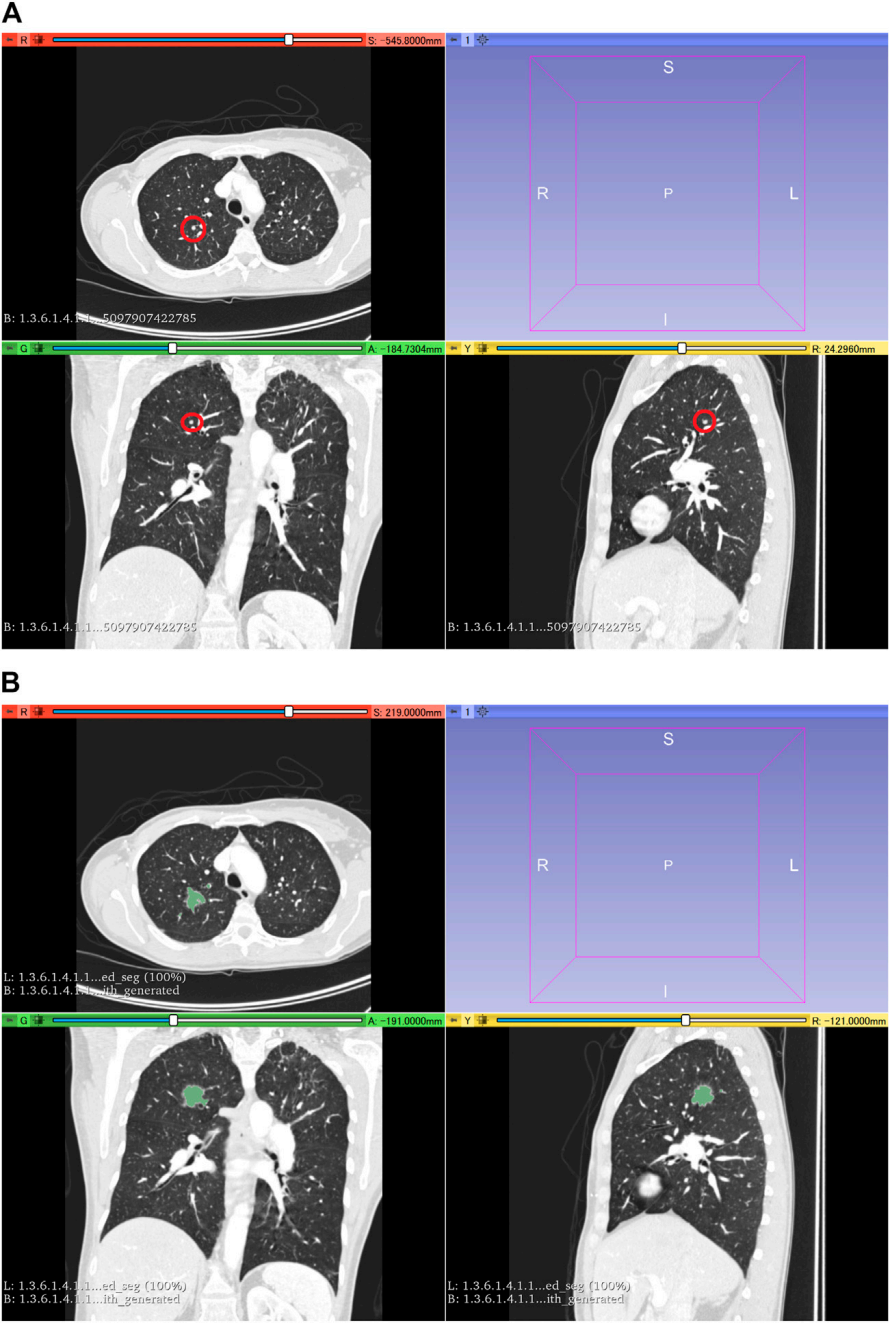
3D CT images of the chest. (A) Original CT images in the LUNA16 dataset. The red circle represents the true nodule specified in the LUNA16 dataset. (B) Lung nodule is artificially generated at the location of the true nodule. Label obtained with the 3D graph cut is superimposed on the 3D CT images.
